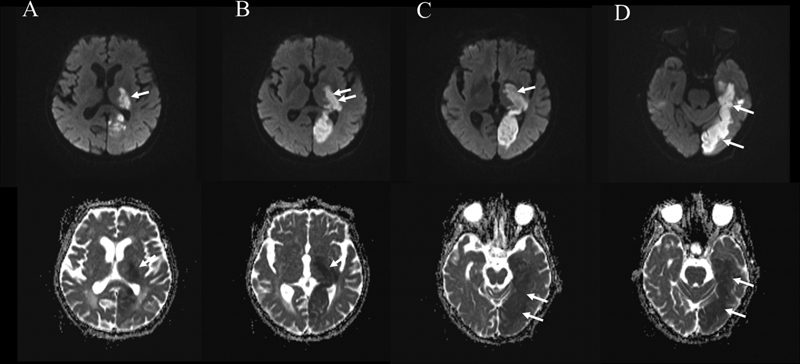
The diffusion-weighted imaging (DWI) and apparent diffusion coefficient (ADC) sequences show the acute diffusion restriction in the vascular territory of the right posterior cerebral artery. The figure shows the lateral portion of the thalamus (
